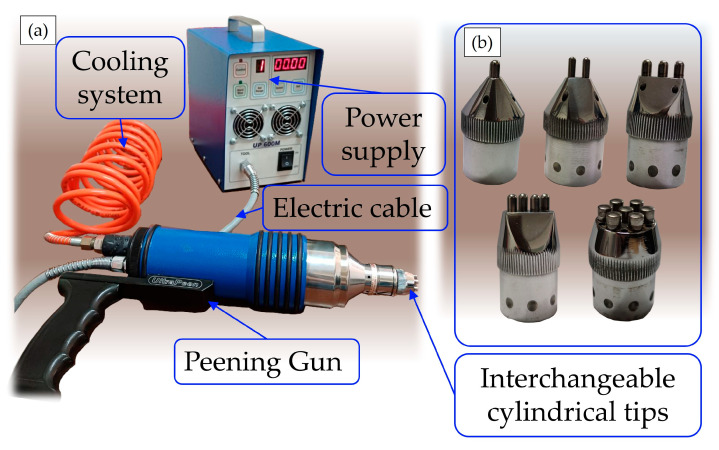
Components for Ultrasonic Peening Treatment: (a) UPT machine; (b) different designs with interchangeable UPT cylindrical tips.
